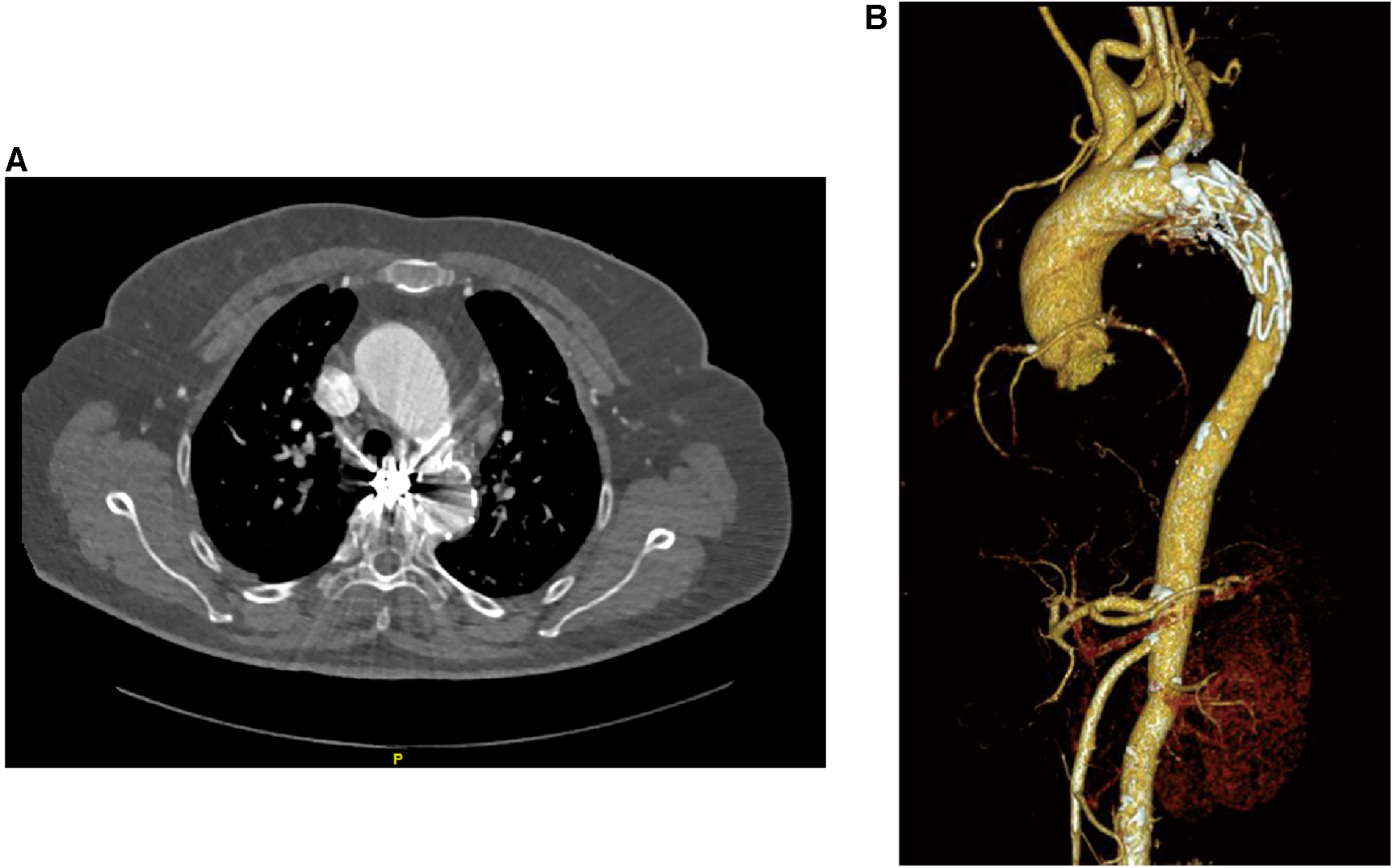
Patient's follow-up examination pictures after 1 year of treatment. (A) CT angiography was performed on the patient 1 year after treatment, and the scan suggests the presence of metallic artifacts from coils within the BAA. (B) Three-dimensional reconstruction of the vessel based on the CT angiography data shows no rupture of the BAA and no new collateral arteries supplying the BAA.
